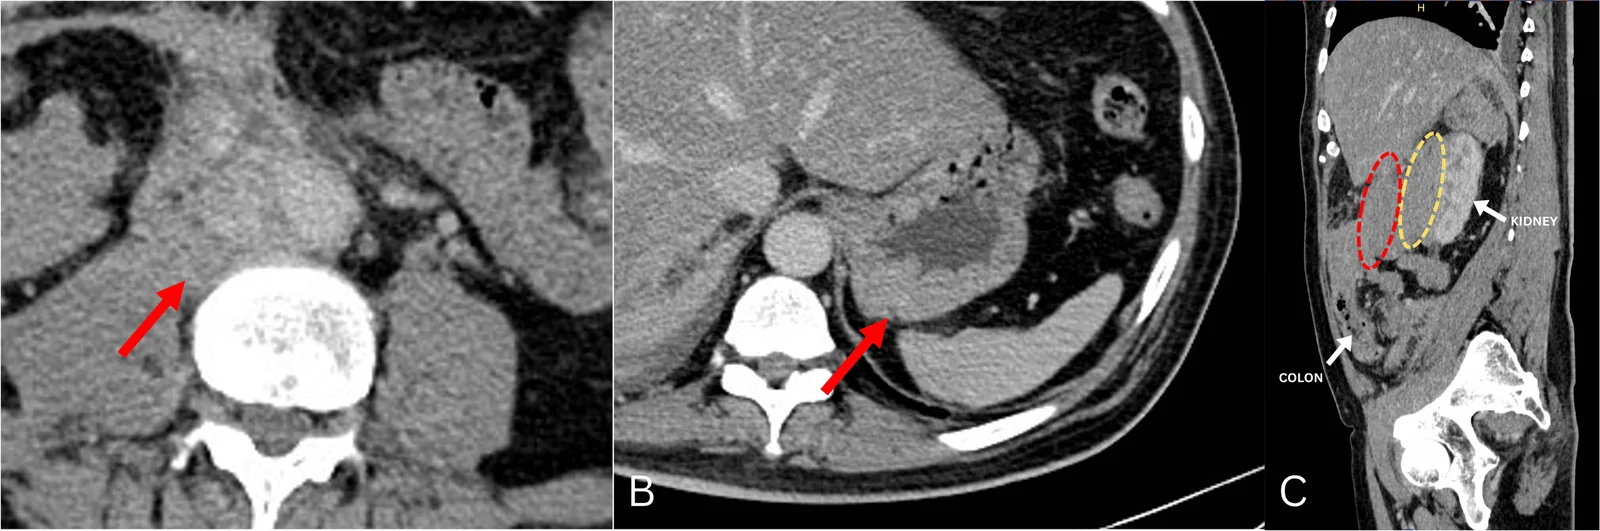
Contrast-enhanced abdominal CT scan A: Retroperitoneal lymphadenopathy with conglomerate formation; B: Asymmetric mural thickening of the greater curvature of the stomach; C: Oblique sagittal image demonstrating a subcapsular renal hematoma (yellow lines) and a heterogeneous hypoenhancing anterior pararenal mass (red lines) extending to the hepatic flexure of the colon
